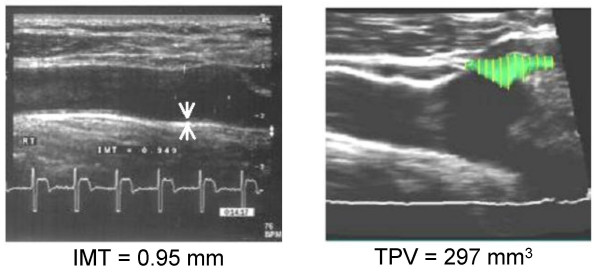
Ultrasound images used for the determination of carotid anatomy in a subject with MetS. The panel on the left shows an image of the right carotid artery used to determine intima media thickness (IMT), with the arrows at the far carotid wall showing where IMT was determined. The panel on the right shows an image used to determine total plaque volume (TPV), with the encircled coloured region defining one of the plaques identified.
